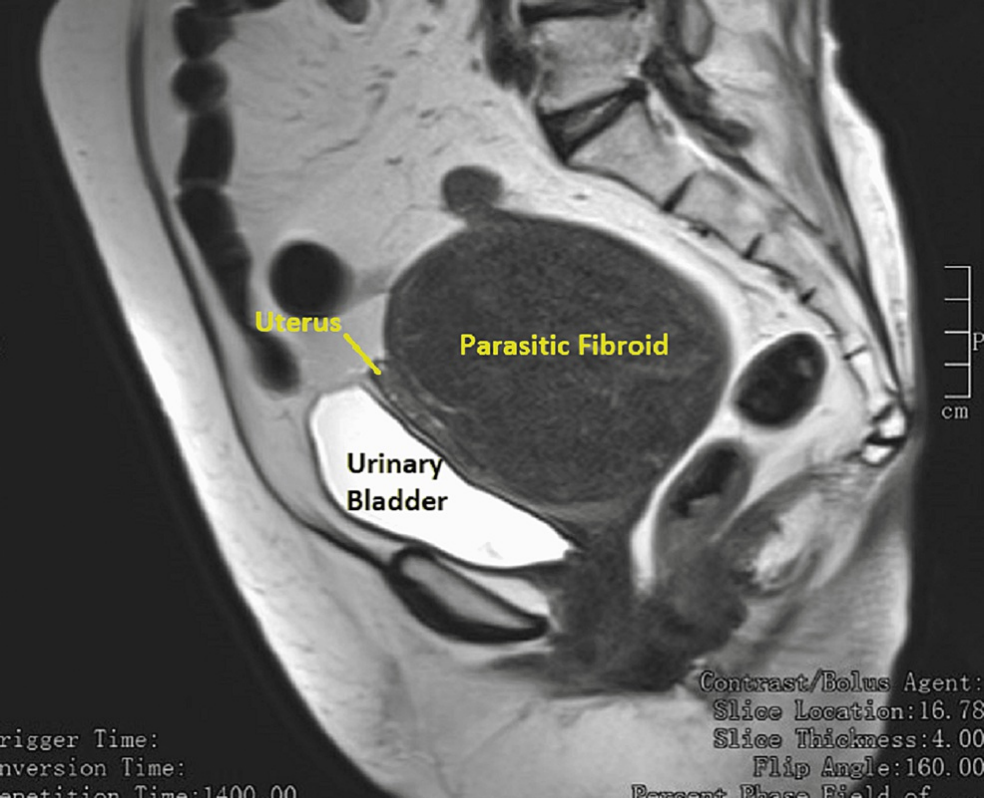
MRI of the abdomen pelvis (T2-weighted sagittal image) showing the relationship between the parasitic fibroid, uterus, and urinary bladder. MRI: magnetic resonance imaging
